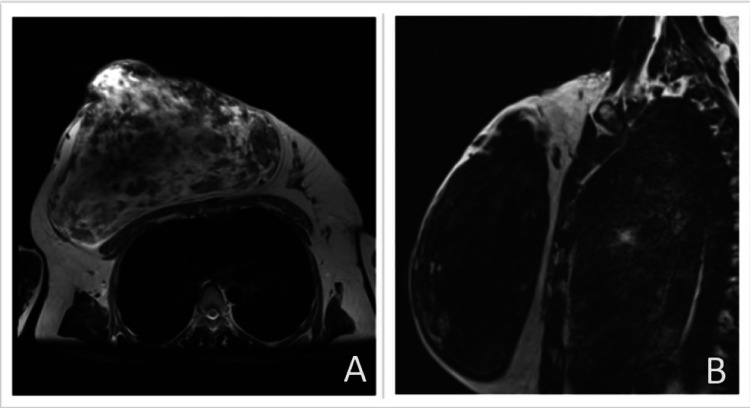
MRI image showing a well-defined, lobulated, heterogeneous lesion of size 15.3 x 21 x 26 cm containing both fat and soft-tissue density. Image A shows an axial view of a thin, radiopaque pseudocapsule suggestive of a fibroadenolipoma (hamartoma) in the left breast, depicting a breast-within-a-breast appearance. Image B shows a sagittal view of the right breast
